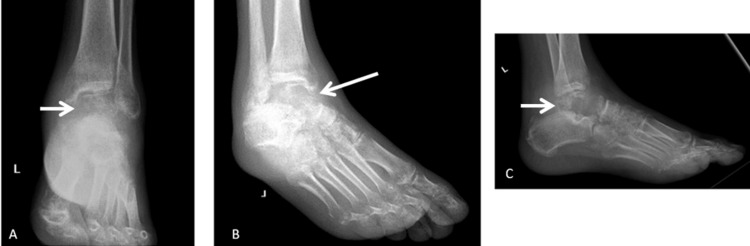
X-ray images of the patient at the time of hospital admission: (A) AP view of the ankle, (B) mortise view of the ankle, and (C) lateral view of the ankle. The white arrows indicate the area of the lesion. AP: anterior-posterior
